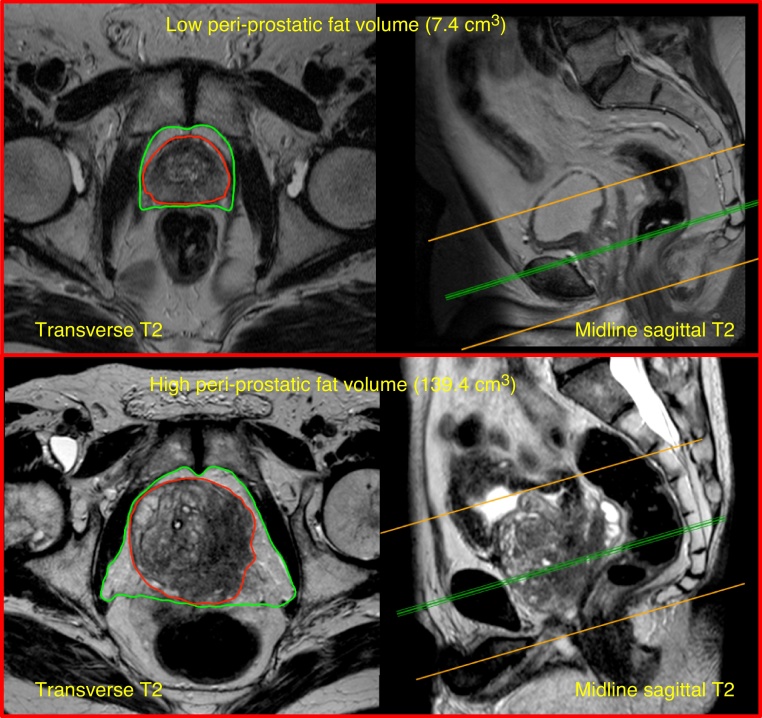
Pelvic magnetic resonance imaging scans of the lowest and highest peri-prostatic fat volume (PPFV) cases. Examples of T2-weighted pelvic magnetic resonance imaging scans of the lowest PPFV (7.4 cm^3^) and highest PPFV (139.4 cm^3^) cases within our cohort. Transverse (with regions of interest marked) and midline sagittal views (with cross-section reference line) are shown with the prostate highlighted in red and the surrounding peri-prostatic fat in green.
